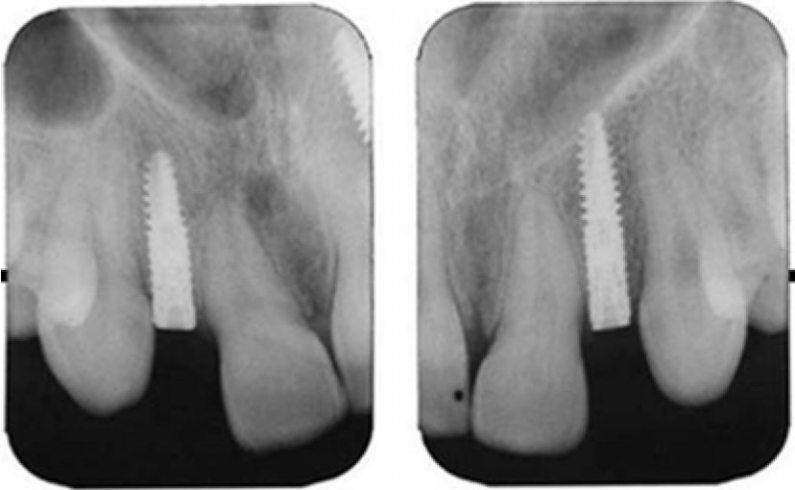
Periapical radiographs after osseointegration
